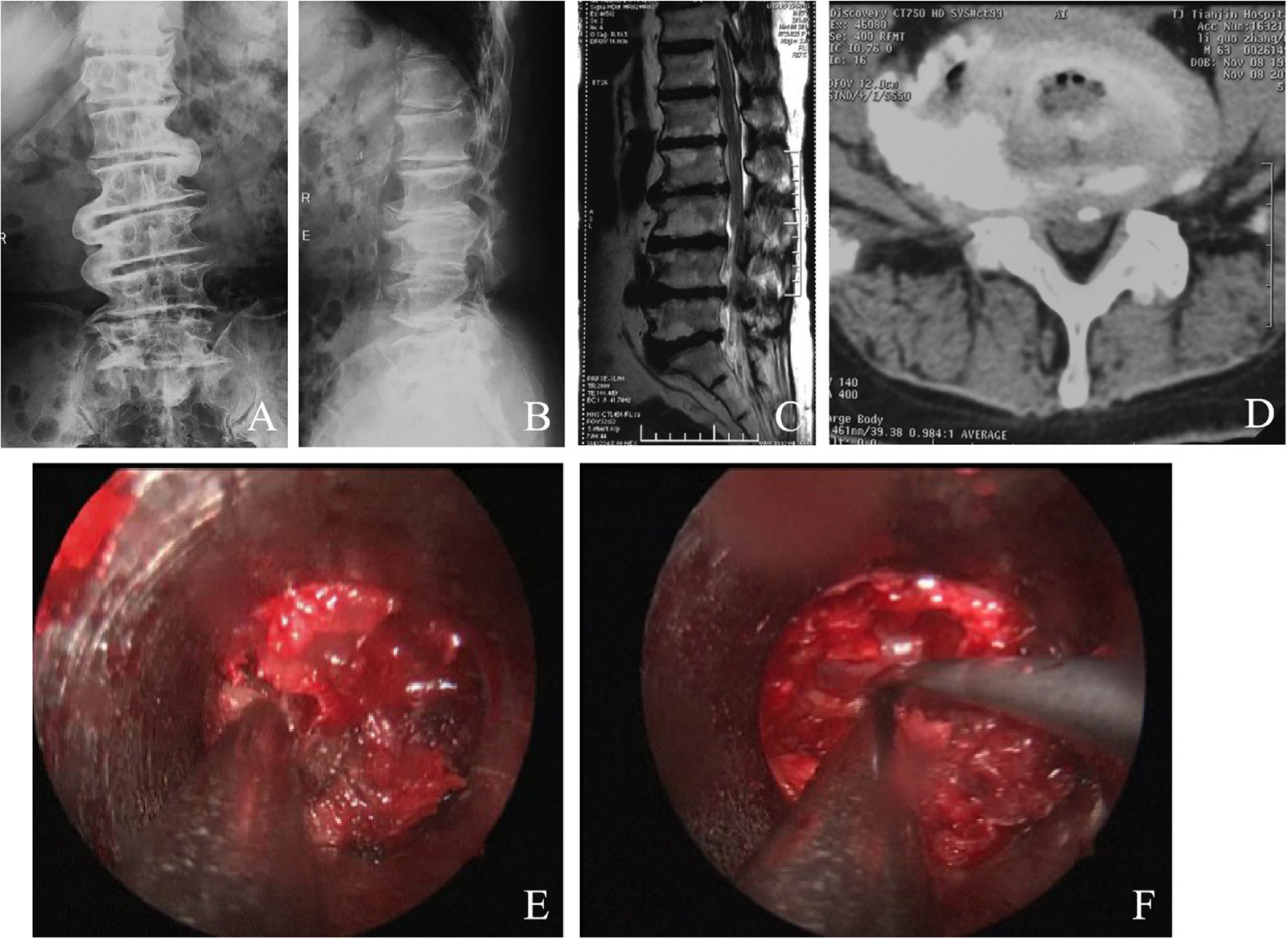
Case 2, a male patient aged 63 years, with lumbar spinal stenosis in the L_3–5_ space. (A‒B) Preoperative anteroposterior and lateral X-Ray revealed severe lumbar vertebral osteoporosis. (C‒D) Preoperative MRI revealed LIDH and severe lumbar spinal stenosis in the L_3–5_ space. PIED was performed first. The anatomic structure was not clearly visualized, with loss of orientation. This patient was intraoperatively shifted to MMED with adequate decompression, loosening of the dural sac and nerve root.
